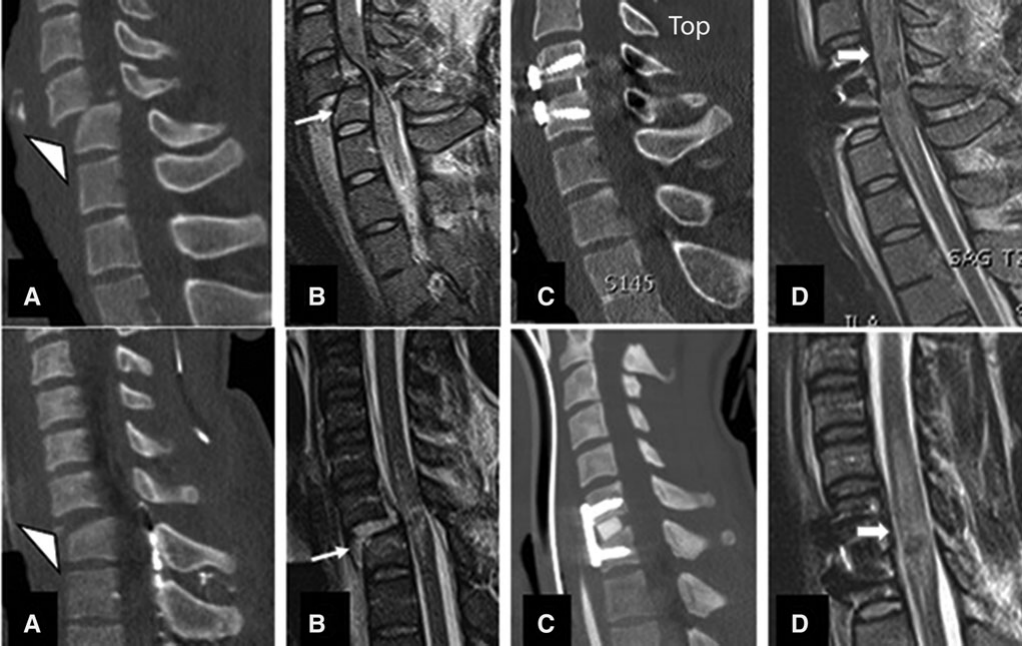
Midsagittal CT and MRI images of two subaxial cervical spine injuries without (upper rows) and with (lower rows) success in decompression. In the upper row a translation rotation injury (A and B, arrowhead and arrow, respectively) was managed with a single-level ACDF, while the lower row indicates another translation rotation and compression fracture (A and B) managed with a single-level of anterior cervical discectomy and fusion (C) and successful decompression (arrow).
